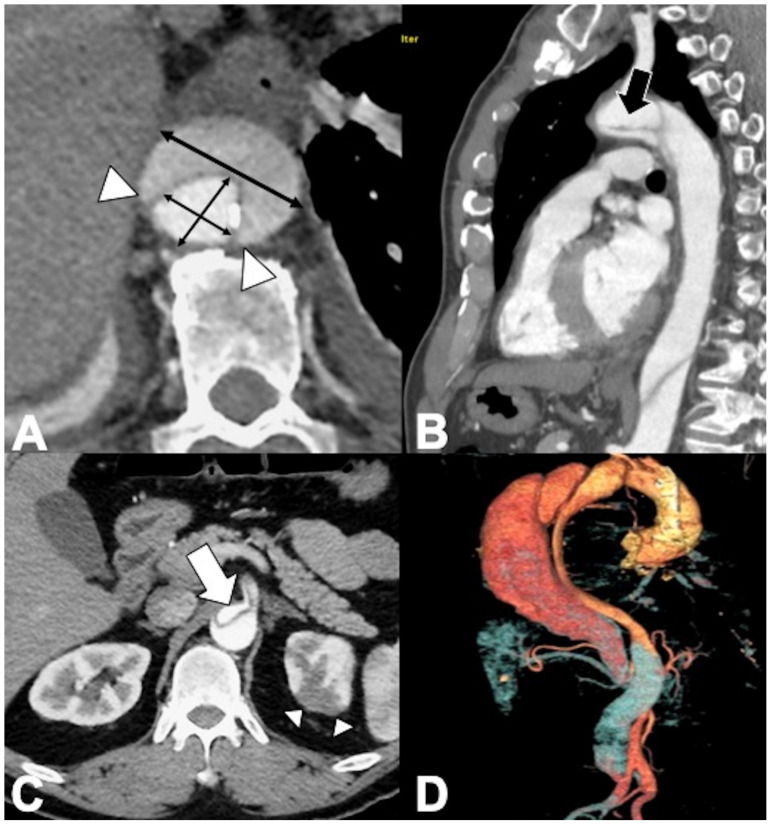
CTA findings in classic dual-barrel aortic dissection. (A) Axial CTA images showing the “beak sign” (arrowheads) in the false lumen because its wall and intimomedial flap usually form acute angles resembling a bird’s beak. In the radiological report, maximum aortic diameter and minimum and maximum aortic true lumen diameter should be included. (B) Sagittal oblique MPR image of non-A-non-B dissection with descending-entry and retrograde arch extension (arrow) in a 75-year-old male presenting with acute chest pain. (C) Axial CTA images. Static aortic dissection is seen as protrusion of the intimal flap (arrow) into the ostium of the affected branch vessel causing subsequent partial or total thrombosis of the branch vessel with resulting perfusion impairments. Note left kidney partial infarct (arrowheads). (D) CTA oblique coronal VR reconstruction image shows a three lumina aortic dissection.
